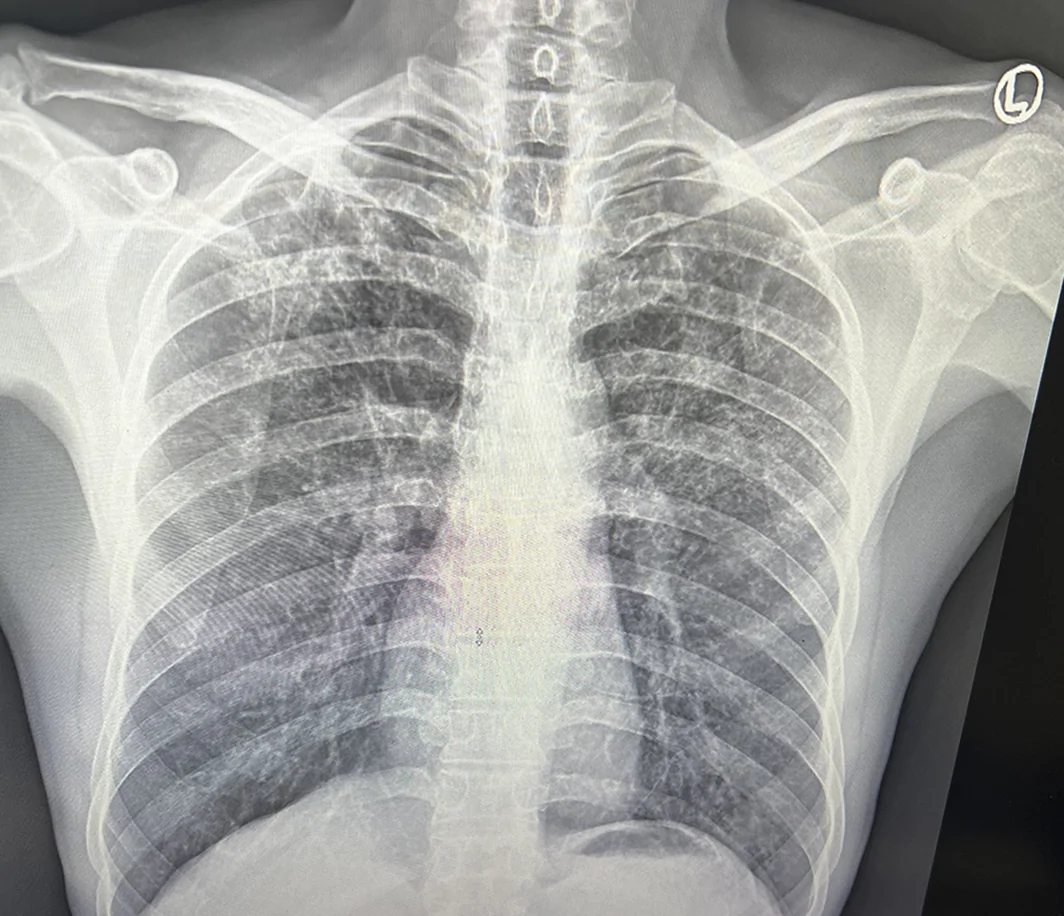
Chest radiograph showing bilateral patchy perihilar and interstitial infiltrates involving the middle and lower lung zones.
